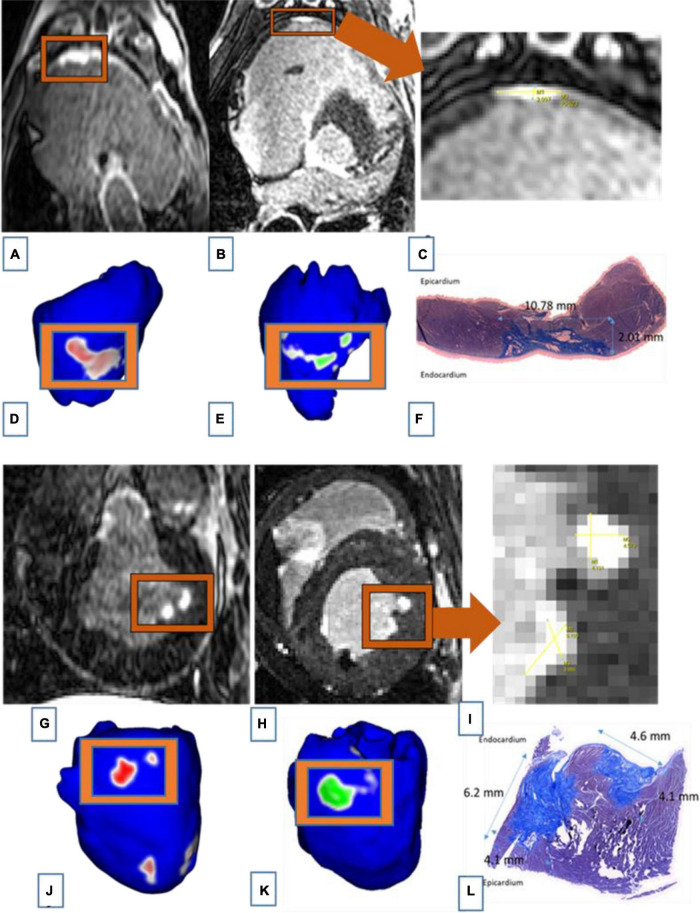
Images of two connected lesions in the RV free wall shown on LGE-MRI and corresponding Corview reconstructions in the acute phase [(A): axial view and (D)] and the chronic phase [(B): axial view and (E)]. Note the similarities between LGE-MRI in the chronic settings (B) and pathological assessment (F). Images of two unconnected lesions in the LV free wall shown on LGE-MRI and corresponding Corview reconstructions in the acute phase [(G): axial view and (J)] and the chronic phase [(H): axial view and (K)]. Note the similarities between LGE-MRI in the chronic settings (H) and pathological assessment (L). Images (C) and (I) are zoomed in figures of B and H, respectively.
